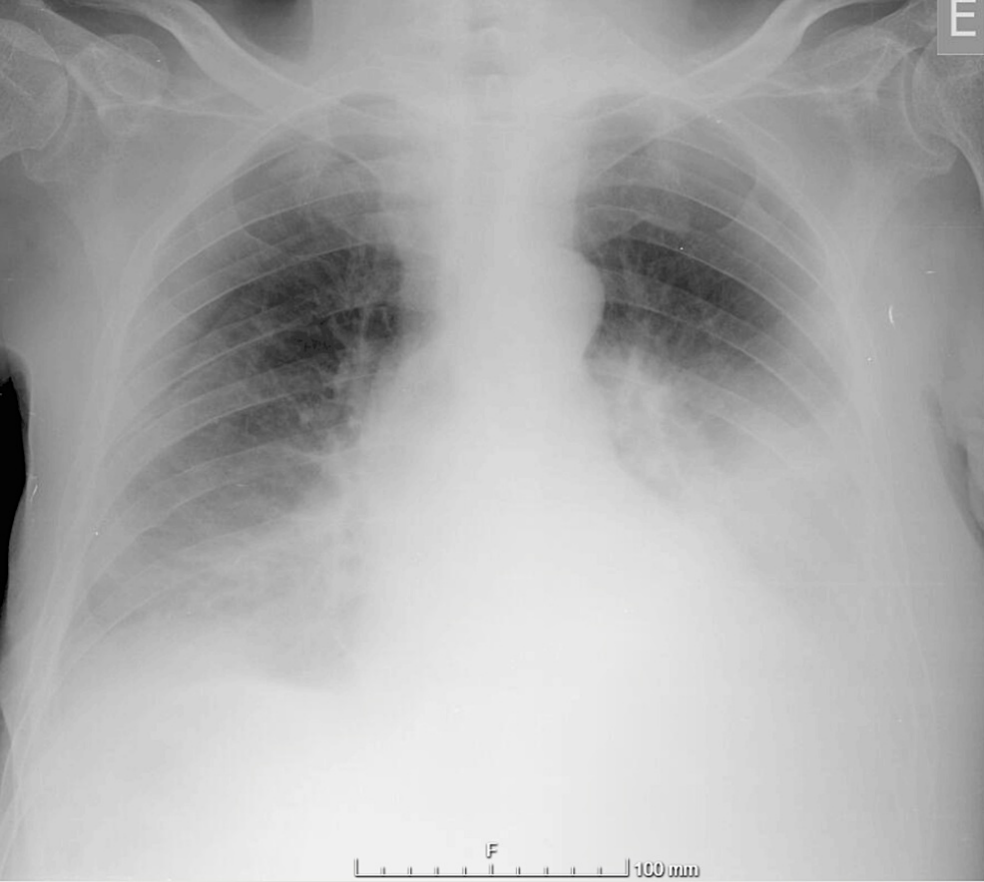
Admission chest X-ray with bilateral pleural effusion
